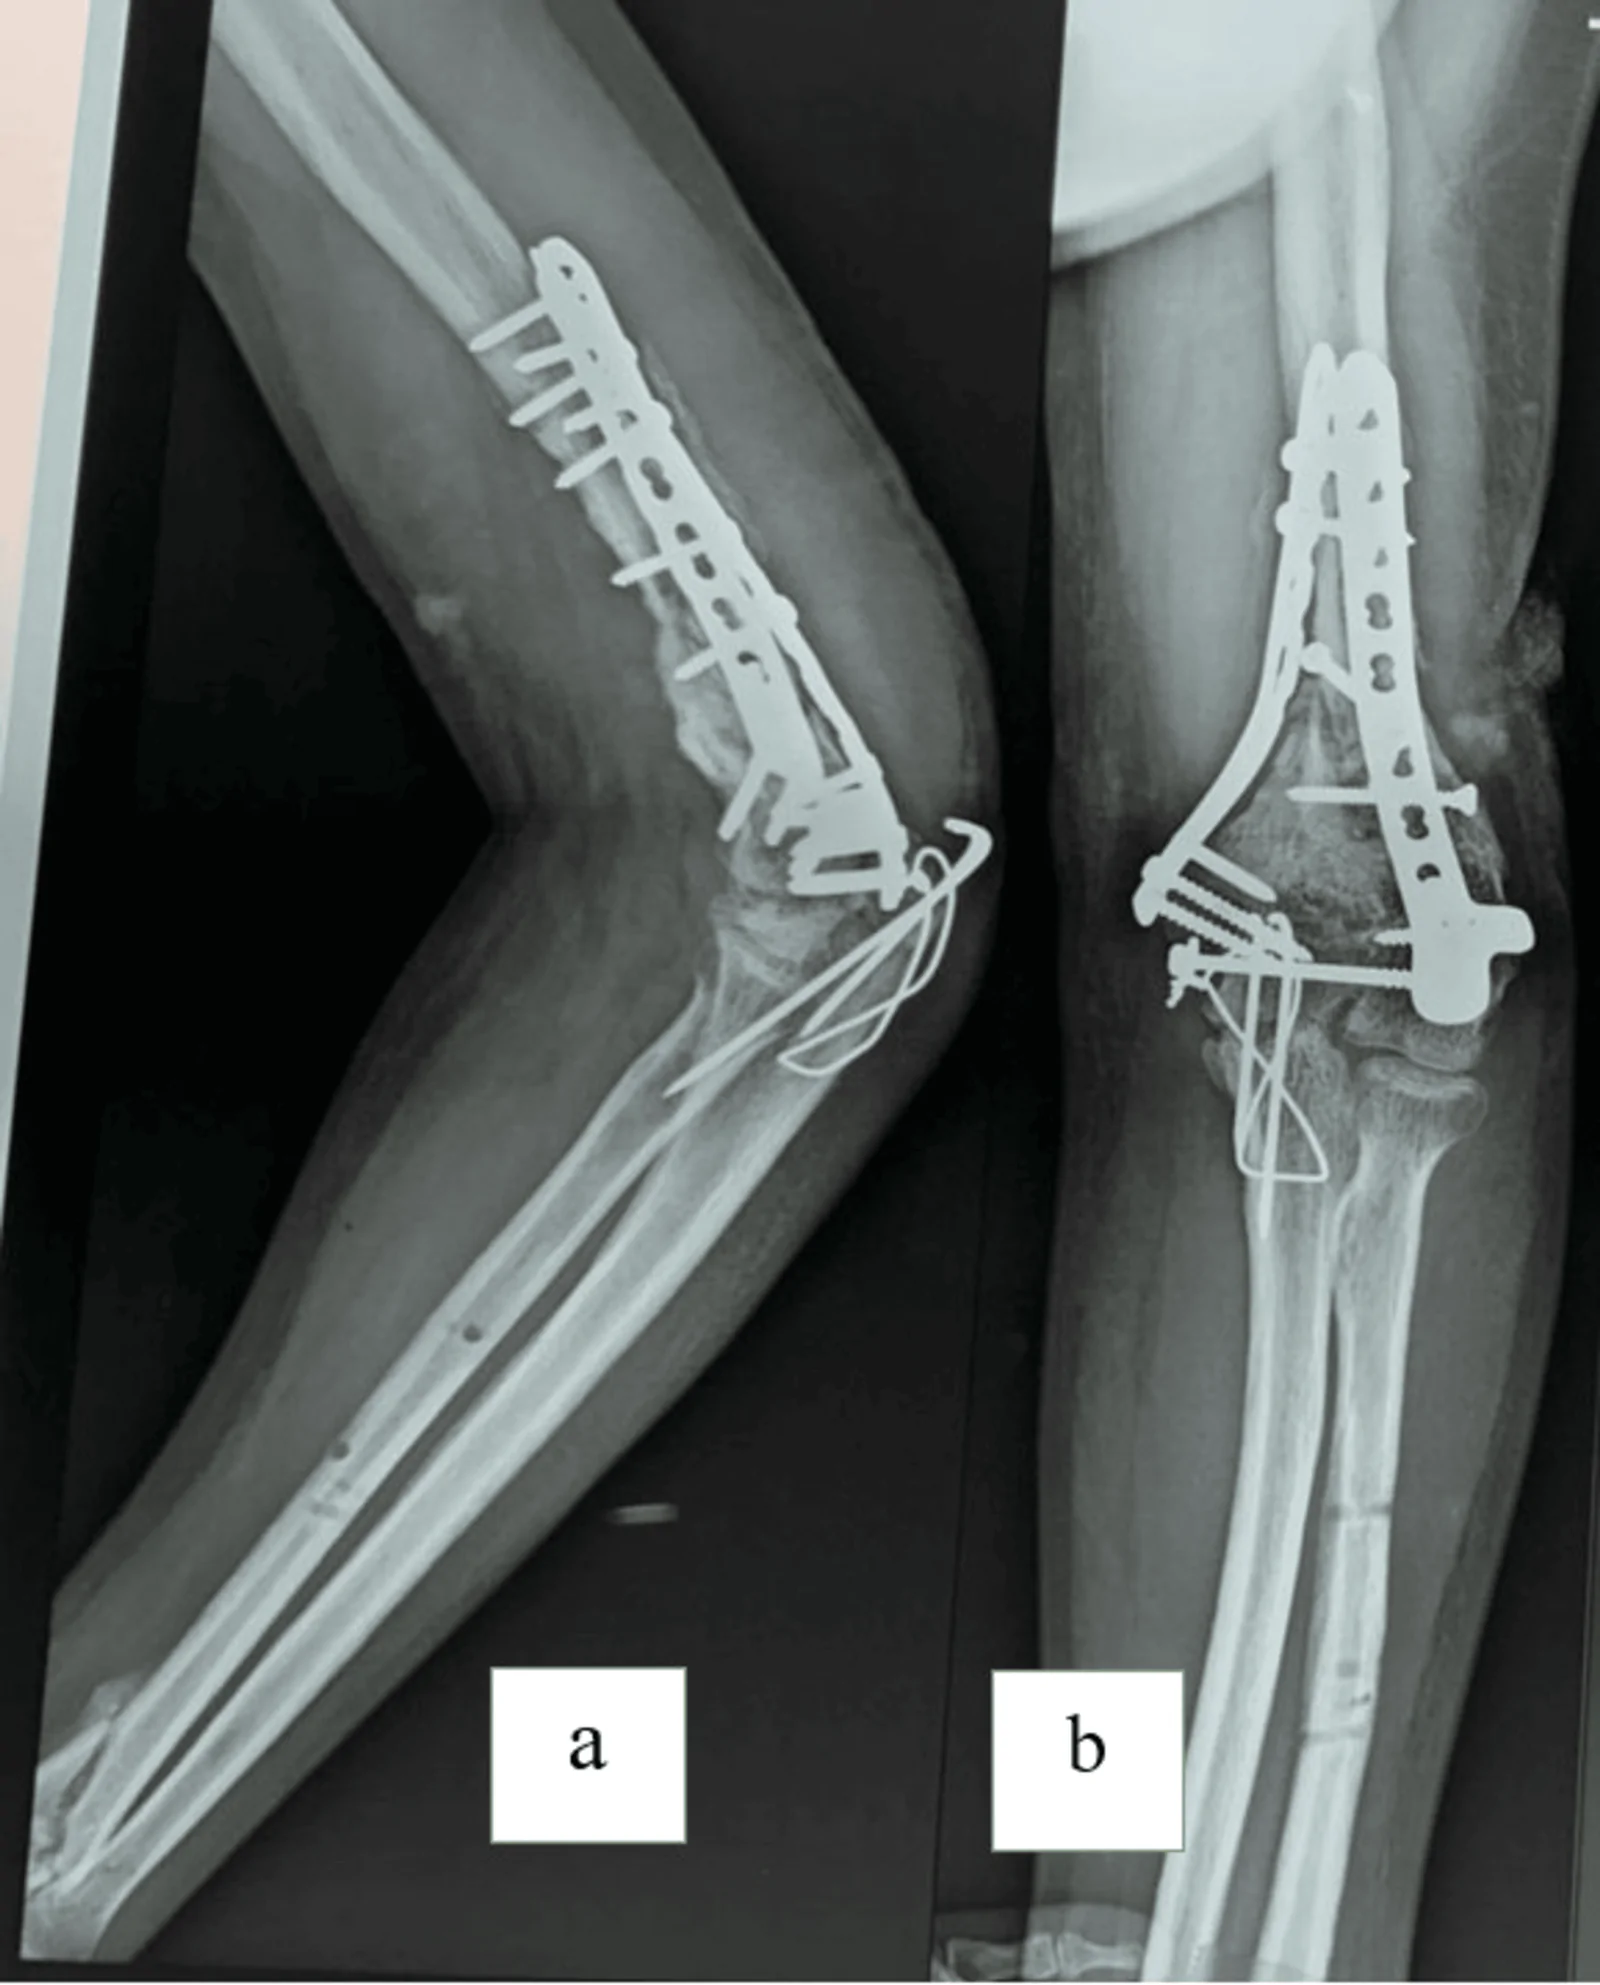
Preoperative X-ray showing distal humerus fracture with implant in situ: (a) lateral view and (b) anteroposterior view
